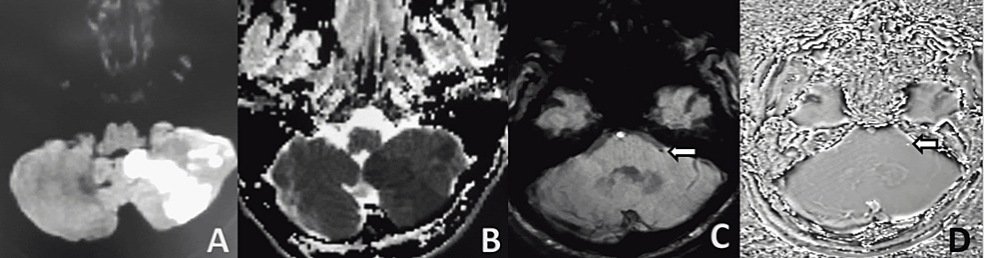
Acute non-haemorrhagic infarct noted in left cerebellar hemisphere showing diffusion restriction on DWI (A) with corresponding low ADC values (B). On magnitude left anterior inferior cerebellar artery (white arrows) appears hypointense (C) appearing bright on phase image (D) suggestive of acute thrombus.
